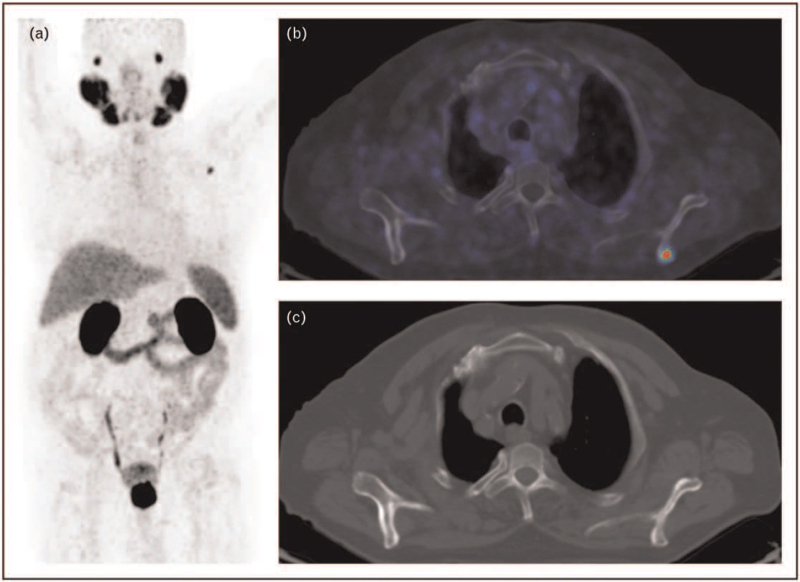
A 80-year-old man with prostate cancer (GS 4+4), previously treated with RT. At the time of the scan, PSA value was 6.4 ng/ml, with ongoing ADT. At the ^68^Ga-PSMA PET/CT scan (a, MIP) a single bone lesion in the left scapula (b, fused image) was found without any alterations on low-dose CT images (c). ^68^Ga-PSMA PET/CT can clarify the site of the disease with unprecedent accuracy and even by detecting lesions without any alteration on CT, thus helping to choose the best therapeutic strategy. ADT, androgen deprivation therapy; CT, computed tomography; MIP, maximum intensity projection; PSMA, prostate-specific membrane antigen.
